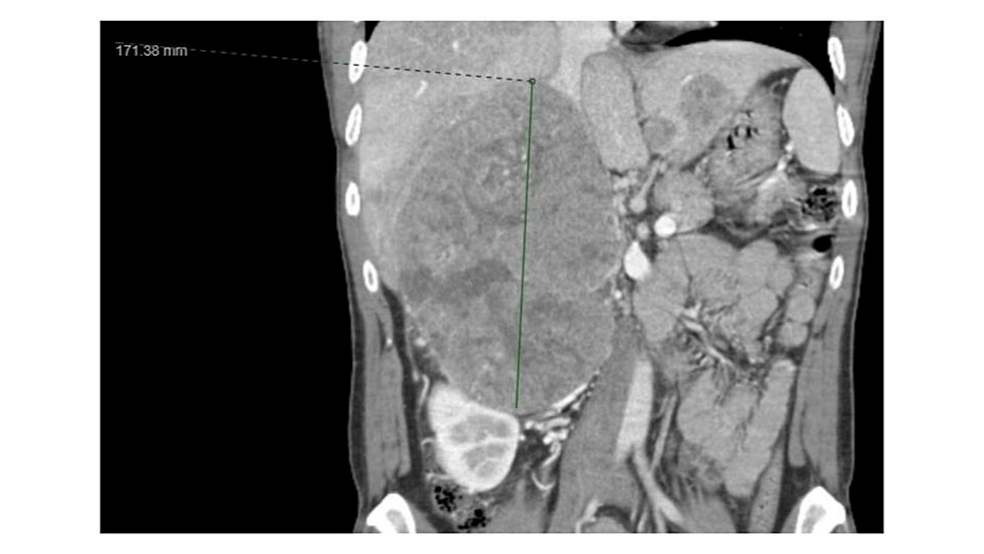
Coronal view of the CT scan demonstrates a large right abdominal heterogeneous enhancing mass measuring 17.1 cm in height.
